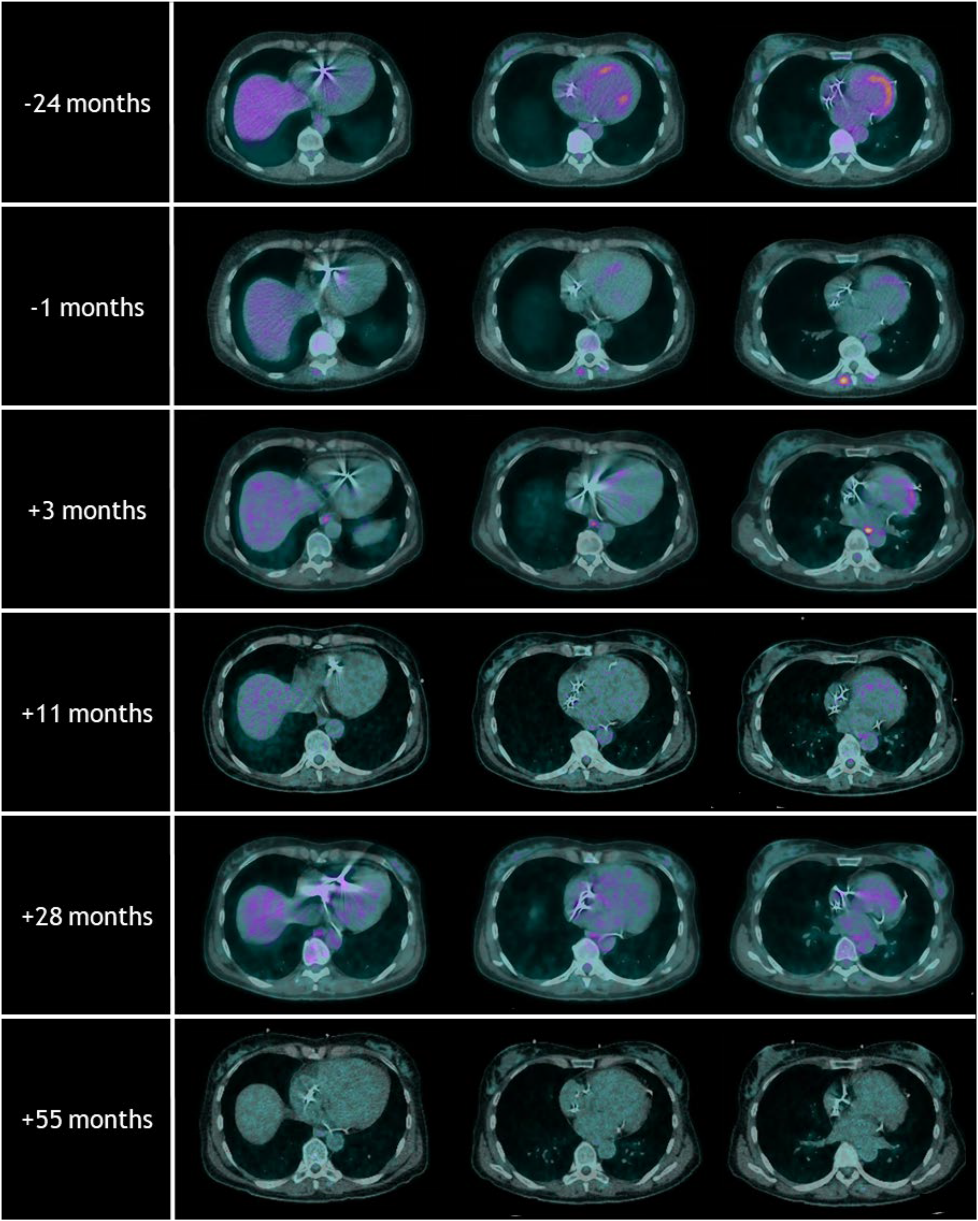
Overview of the repeated [^18^F]FDG-PET/CT scans with the timing relative to radiotherapy treatment. Note the hypermetabolic areas of the anteroseptal segments at the [^18^F]FDG-PET/CT scans prior to and 24 months before radiotherapy. Follow-up [^18^F]FDG-PET/CT scans after radiotherapy showed regression of these local hypermetabolic areas. The [^18^F]FDG-PET/CT scan at 55 months did not show any signs of active cardiac sarcoidosis.
